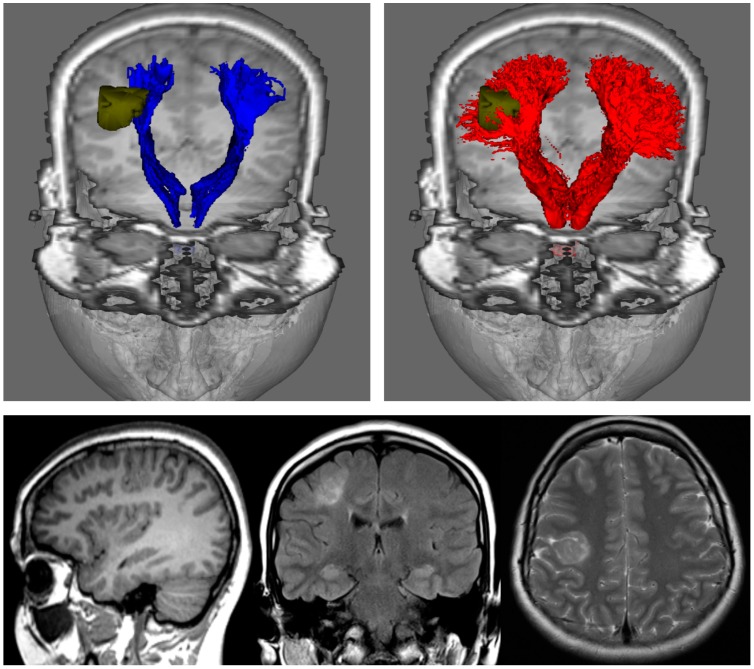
Fibre-tracking results on a 24-year-old woman with a right focal cortical dysplasia situated in the right posterior frontal lobe (see images in bottom row). These results were obtained using a seed region in the brainstem and target regions in the sensorimotor cortices. DTI-based tractography (blue) and CSD-based tractography (red) were derived from the same diffusion MRI data set); segmented pathology volumes (green) overlaid on T1-weighted image. DTI-based tractography suggests that only the medial aspect of the lesion impinges on the corticospinal tracts, whereas CSD-based tractography suggests that the lesion is enveloped by medial and lateral projections. DTI: Diffusion Tensor Imaging; CSD: constrained spherical deconvolution. Note that while only DTI combined with deterministic tracking is shown in the top-left image, the results of combining DTI with probabilistic tracking are expected to be very similar (cf. left and middle images in Figure 2). Figure modified from a figure previously published in [30], with permission of American Association of Neurological Surgeons, 2013.
